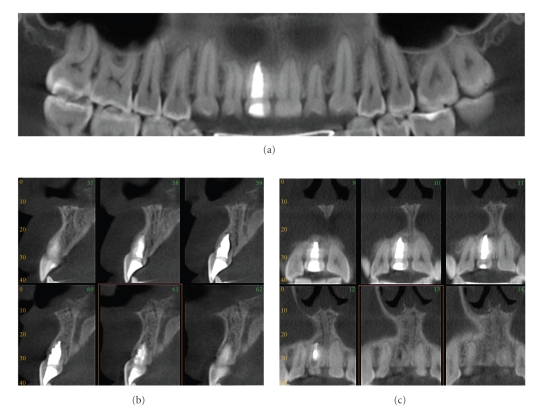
Replacement external resorption associated with root canal filled right maxillary central incisor. An oblique multiplanar reformatted “panoramic” image (a) shows the dentition with minimal restorations and a single root canal filled maxillary right central incisor; note that the obturation is large in relation to the width of the adjacent left maxillary central suggesting endodontic treatment at an early age. Sequential 1 mm cross-sectional (b) and parasagittal (c) images show bone trabecular-like replacement of the superior and palatal aspects of the root indicative of replacement resorption. Initial management consisted of conventional endodontic treatment. Because of the questionable long-term prognosis of the apical resorptive lesions, periodic CBCT imaging is recommended with a view towards surgical revision therapy consisting of apicoectomy and retrograde root canal treatment (data acquired on an iCAT, Imaging Sciences International, Hatfield, PA USA at 0.4 mm resolution and reformatted using InVivo Dental, Anatomage, San Jose, CA).
